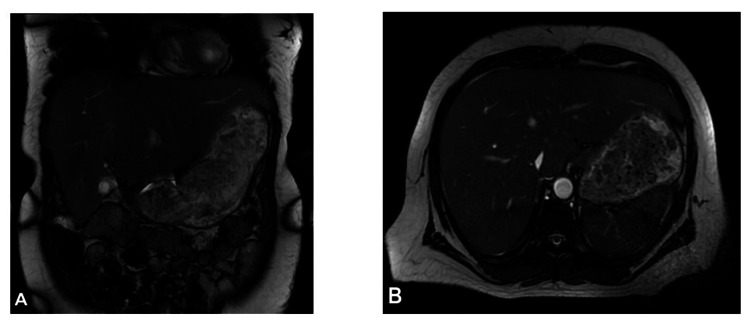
Abdominal magnetic resonance imaging (MRI) findings: (A) There is hepatomegaly with the liver measuring 20.5cm in the craniocaudal dimension (after conservative therapy). (B) A trace amount of perihepatic ascites is present with hepatic and portal veins patent. There is no biliary ductal dilatation, and the gallbladder, spleen, adrenals, and kidneys are within normal limits.
